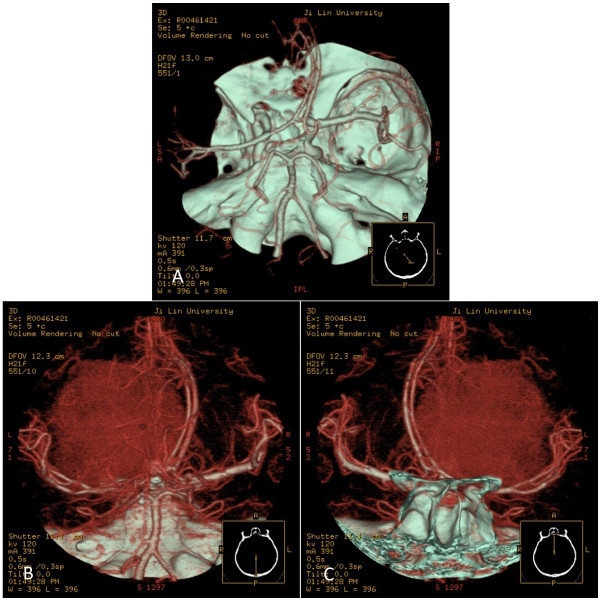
CTA of the brain showing the association of the tumor with adjacent vascular structures. A: Axial computed tomography angiography (CTA). The tumor compressed the ipsilateral anterior and middle cerebral artery (arrows). B-C: CTA image. The tumor was fed by branching arteries from the middle and anterior cerebral arteries.
